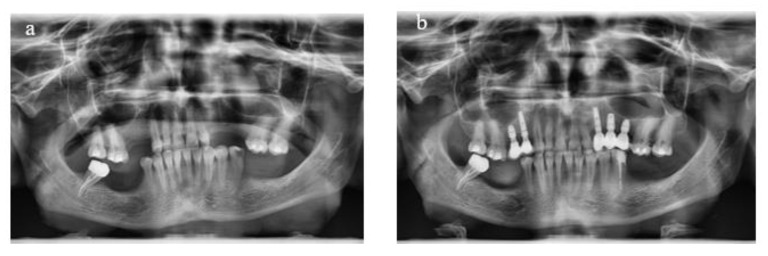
Panoramic radiographs taken before the treatment to assist in diagnosis and treatment planning (a) and following implant placement and prosthesis delivery (b).
